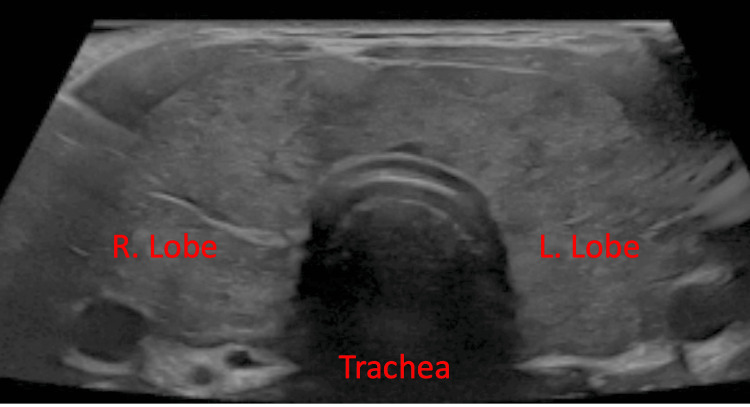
Thyroid ultrasound showing diffuse symmetric enlargement of the thyroid gland including isthmus. Right (R) thyroid gland measured 6 x 2.3 x 2.9 cm and left (L) thyroid gland measured 5.8 x 2.3 x 2 cm. Ultrasound findings also suggested bilateral reactive cervical lymph nodes and increased vascularity.
